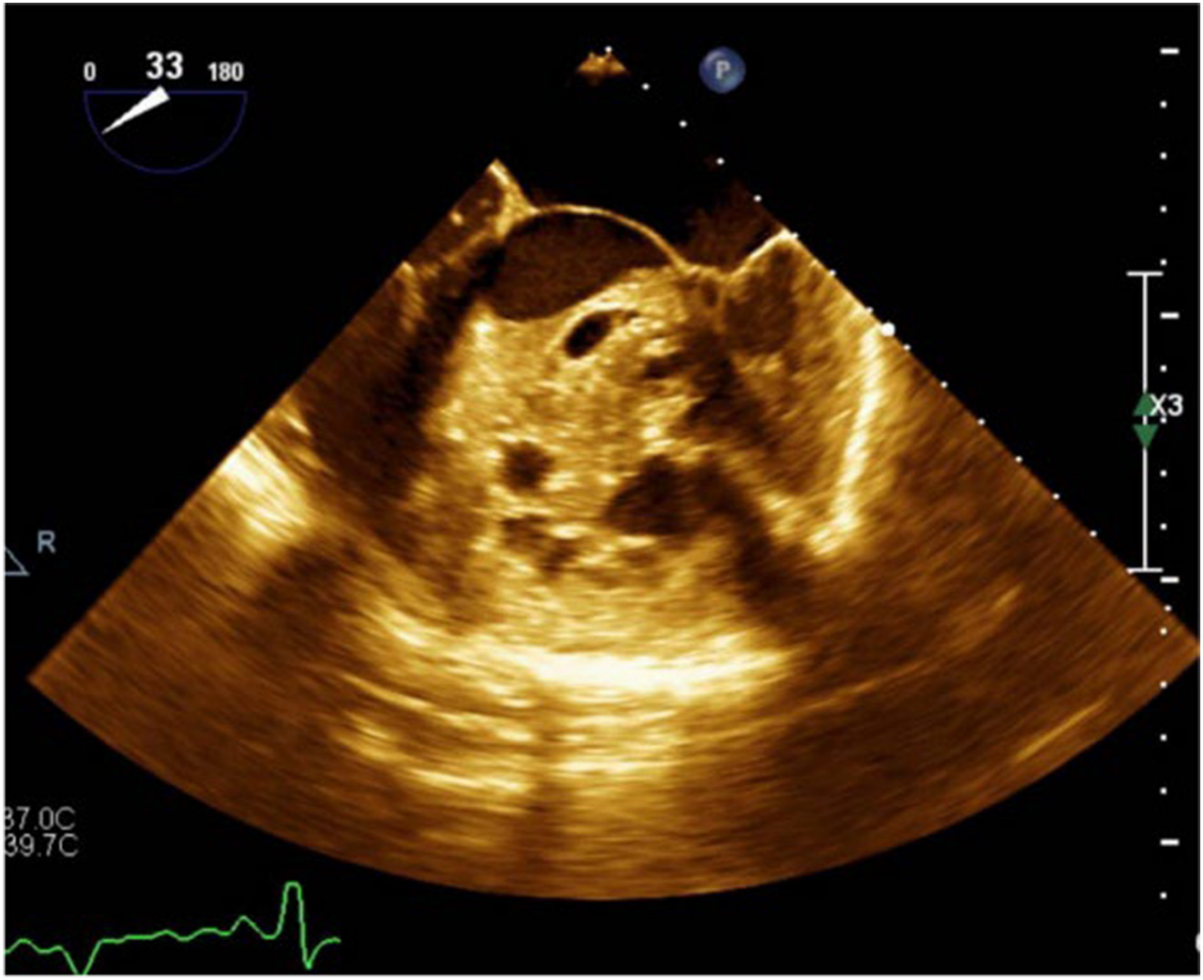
TEE of LV cavity of a 35‐year‐old male with multi‐organ hydatid cyst. TEE shows a Large multi‐lobulated and multi‐cystic mass with septations in some of the cysts and a water‐lily pattern in at least two cysts attached to the septum. LV, left ventricle; TEE, transesophageal echocardiography.
